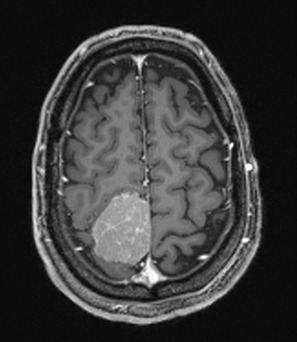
Axial view magnetic resonance image of the brain.
